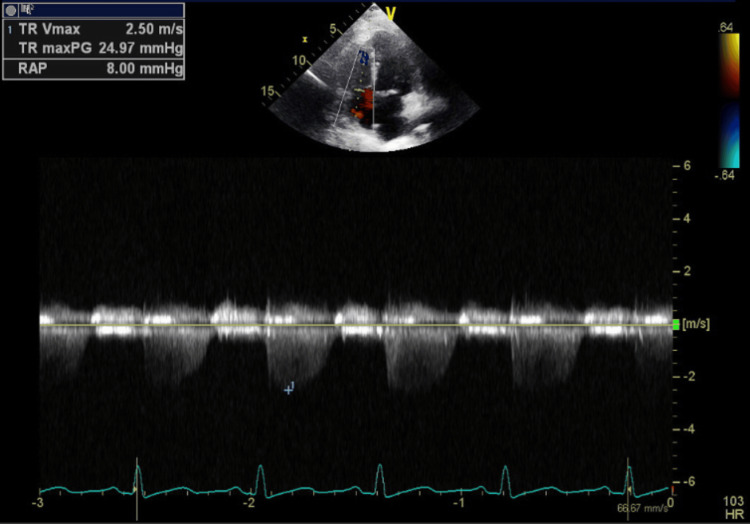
Transthoracic echocardiography - image 4 Transthoracic echocardiography on admission; apical 4-chamber view measuring TR Vmax at 2.50 m/s and RAP at 8 mmHg
